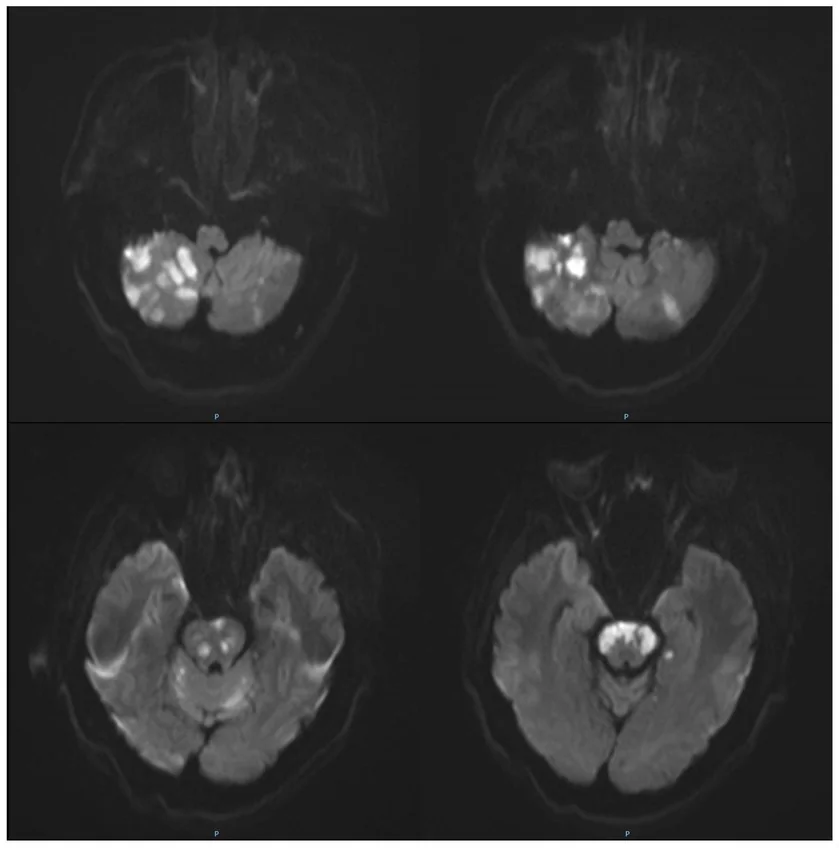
The diffusion weighted imaging (DWI) of the head on January 10, 2023. Brainstem, bilateral cerebellar hemispheres, left hippocampal region, the periventricular region adjacent to the posterior horn of the left lateral ventricle, and right thalamus showed punctate hyperintense lesions.
